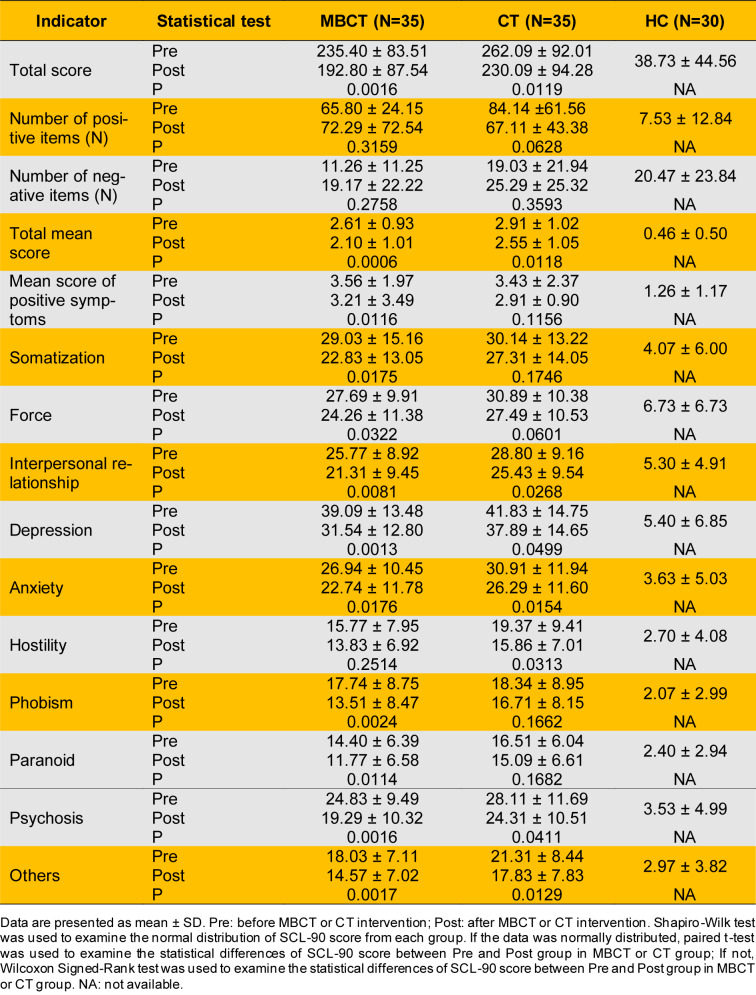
SCL-90 score of all patients with depression received MBCT or CT
